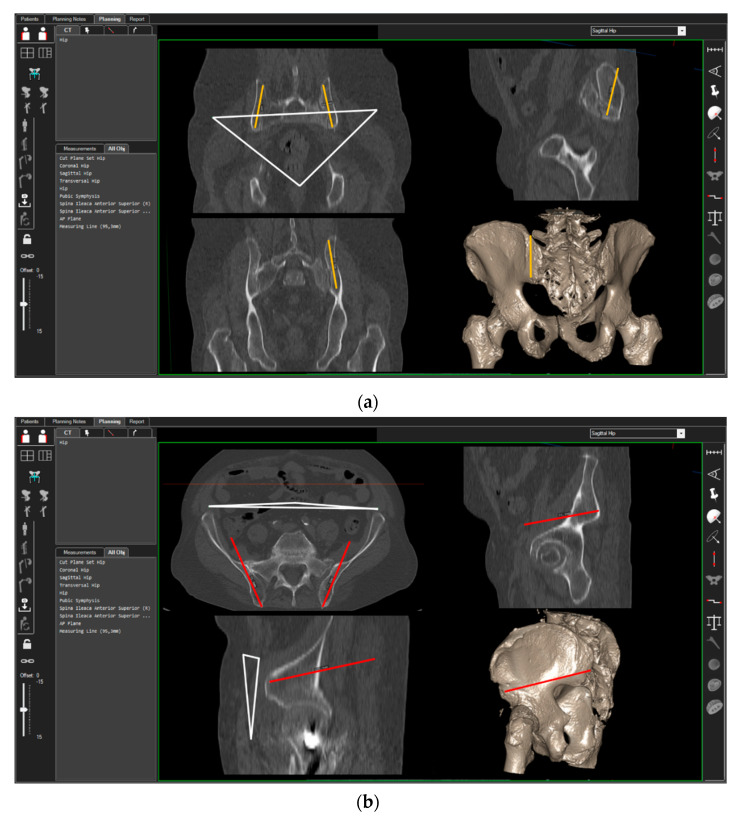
Setting of the 3D-CT-based measurement of the ilium screws in cranio-caudal (a) and dorso-ventral (b) orientation. White triangle = anterior pelvic plane (APP); orange line = position of the TIFIcc, red line = position of the TIFIdv.
